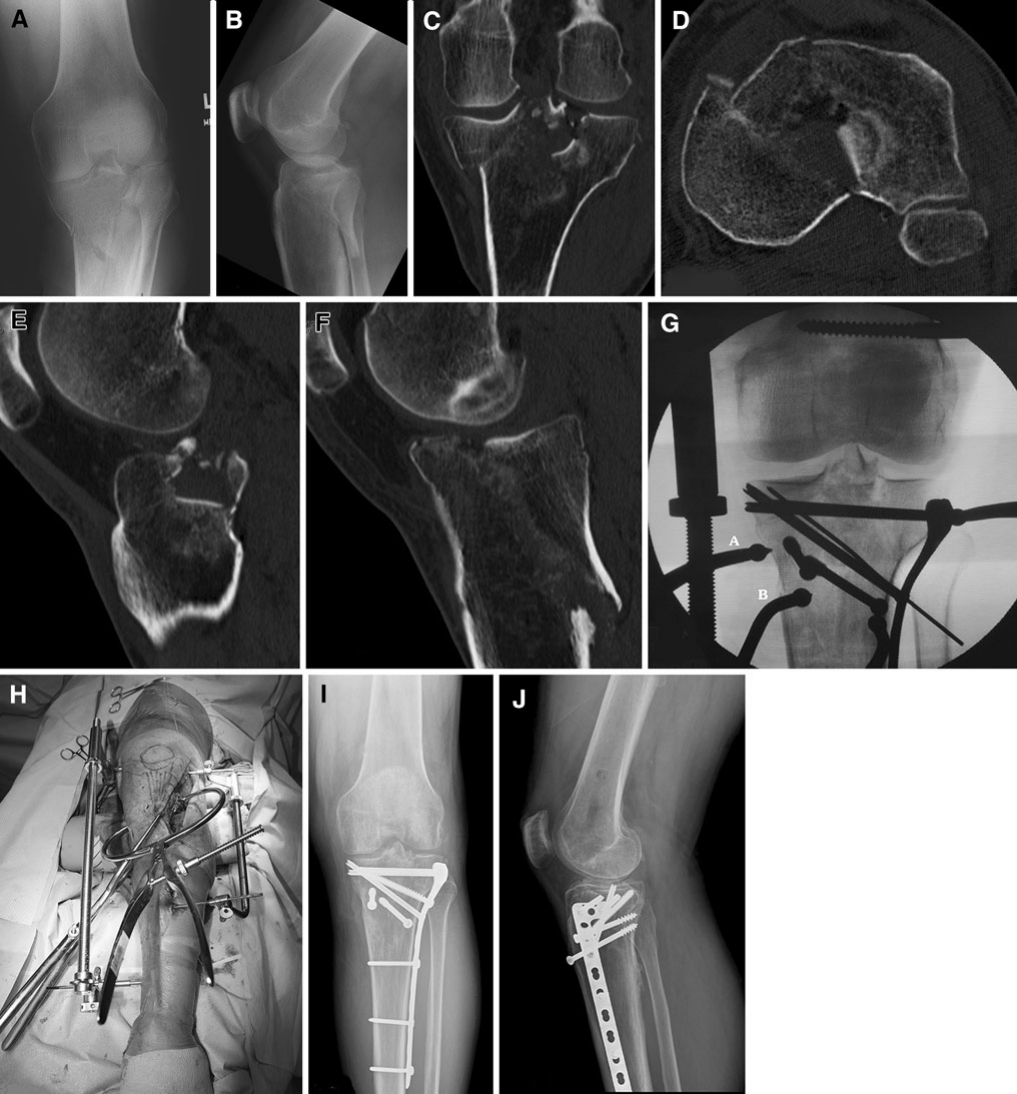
(A) Preoperative AP radiograph of a posterior plateau fracture with involvement of posteromedial, posterocentral, and posterolateral left tibial plateau. Anterolateral joint line is intact. (B) Preoperative lateral radiograph showing extent of posterior plateau articular displacement and intact anterior plateau cortical bone. (C) Coronal CT image showing posteromedial plateau fragment, comminution of tibial eminence, and posterolateral articular fracture depression. Intact anterolateral plateau is present. (D) Axial CT image anterior and posterior medial plateau fractures, 90° rotated posterolateral joint fragment, and intact anterolateral cortical margin of the left tibial plateau. (E) Centrolateral sagittal CT scan revealing substantial posterior depression of articular cartilage with intact anterior half of plateau cortical bone. (F) Sagittal CT scan of medial tibial plateau showing a large posteromedial plateau fragment and oblique major fracture line. (G) AP fluoroscopic view of left tibial plateau fracture reduction technique. Ball-tipped clamp A compresses the lateral plate to osteotomized Gerdy’s fragment and lateral plateau bone. Ball-tipped clamp B reduces posteromedial plateau oblique articular fragment through percutaneous insertion. Two separate AP lag screws are placed into the posteromedial plateau. (H) Clinical appearance of the use of intraoperative bilateral transarticular coronal plane distractors (medial and lateral), anterolateral incision, and the use of the periarticular tong reduction clamps. (I) AP radiographs showing complete healing and axis alignment with anatomic reduction of joint surfaces 3 years after fracture. (J) Lateral radiograph of healed left plateau fracture showing reduction of posterior fractures fragments, restoration of plateau joint surfaces, and position of hardware.
